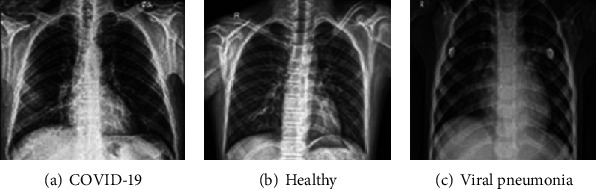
Samples of X-ray images used in this study.
